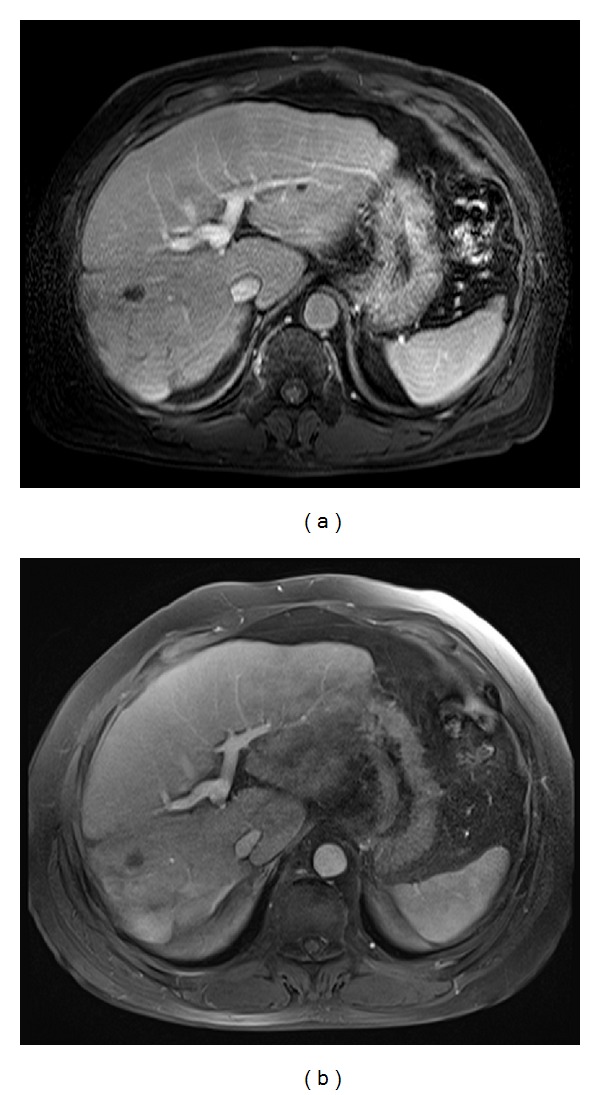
MR images in a 65-year-old man with liver cirrhosis. The conventional breath-hold T1-weighted 3D GRE sequence (acquisition time: 23 seconds) and the CAIPIRINHA 3D GRE sequence (acquisition time: 12 seconds) were obtained during the early hepatic venous phase. T1-weighted 3D GRE (a) shows blurred resolution of the intrahepatic vessel and the hepatic margin caused by motion artifacts. Much greater sharpness of the hepatic edge and a clearer depiction of the intrahepatic vessel are shown on the T1-weighted 3D CAIPIRINHA GRE sequence.
